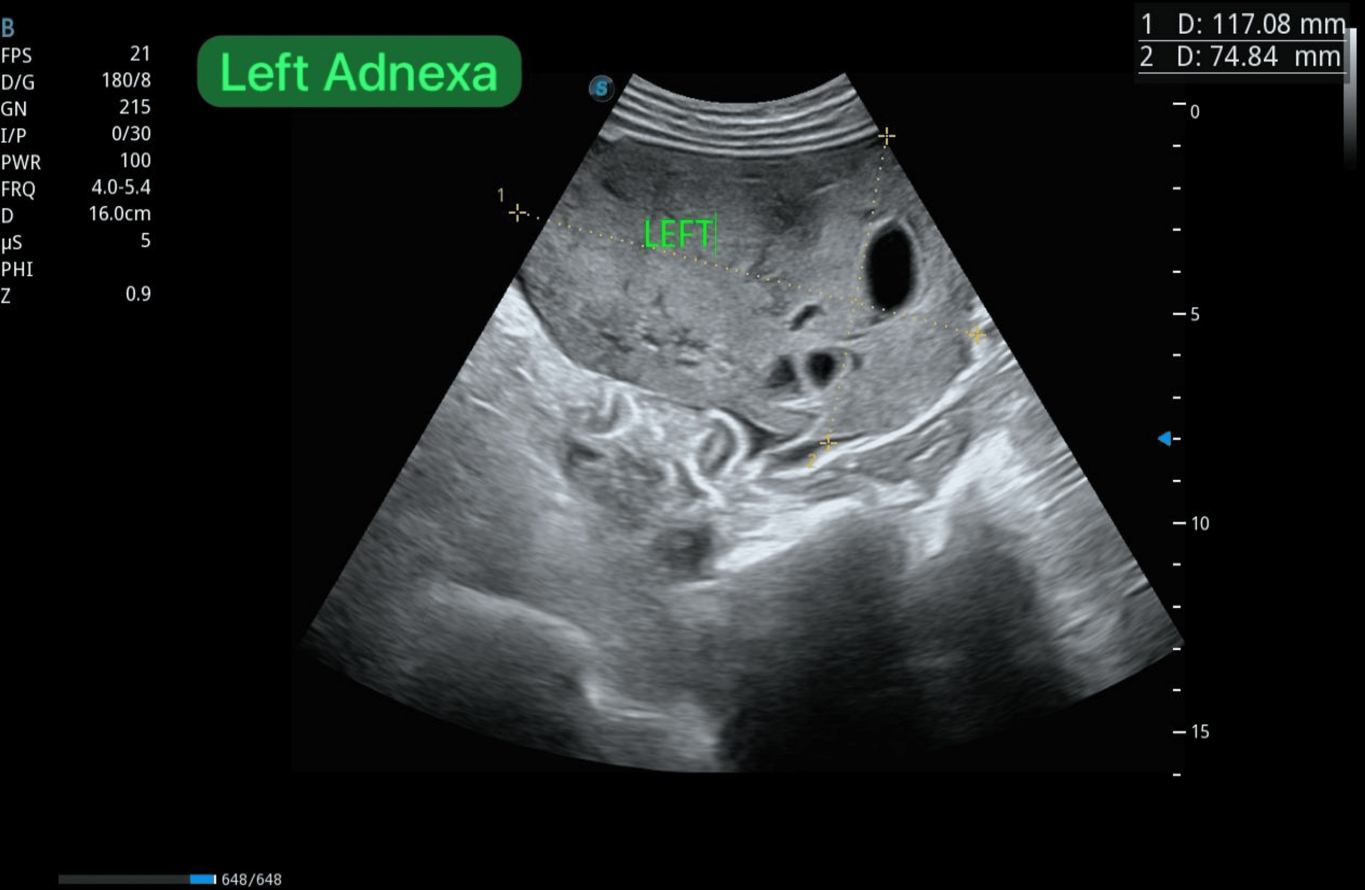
Transabdominal ultrasound of the left adnexa revealing a large complex adnexal mass
